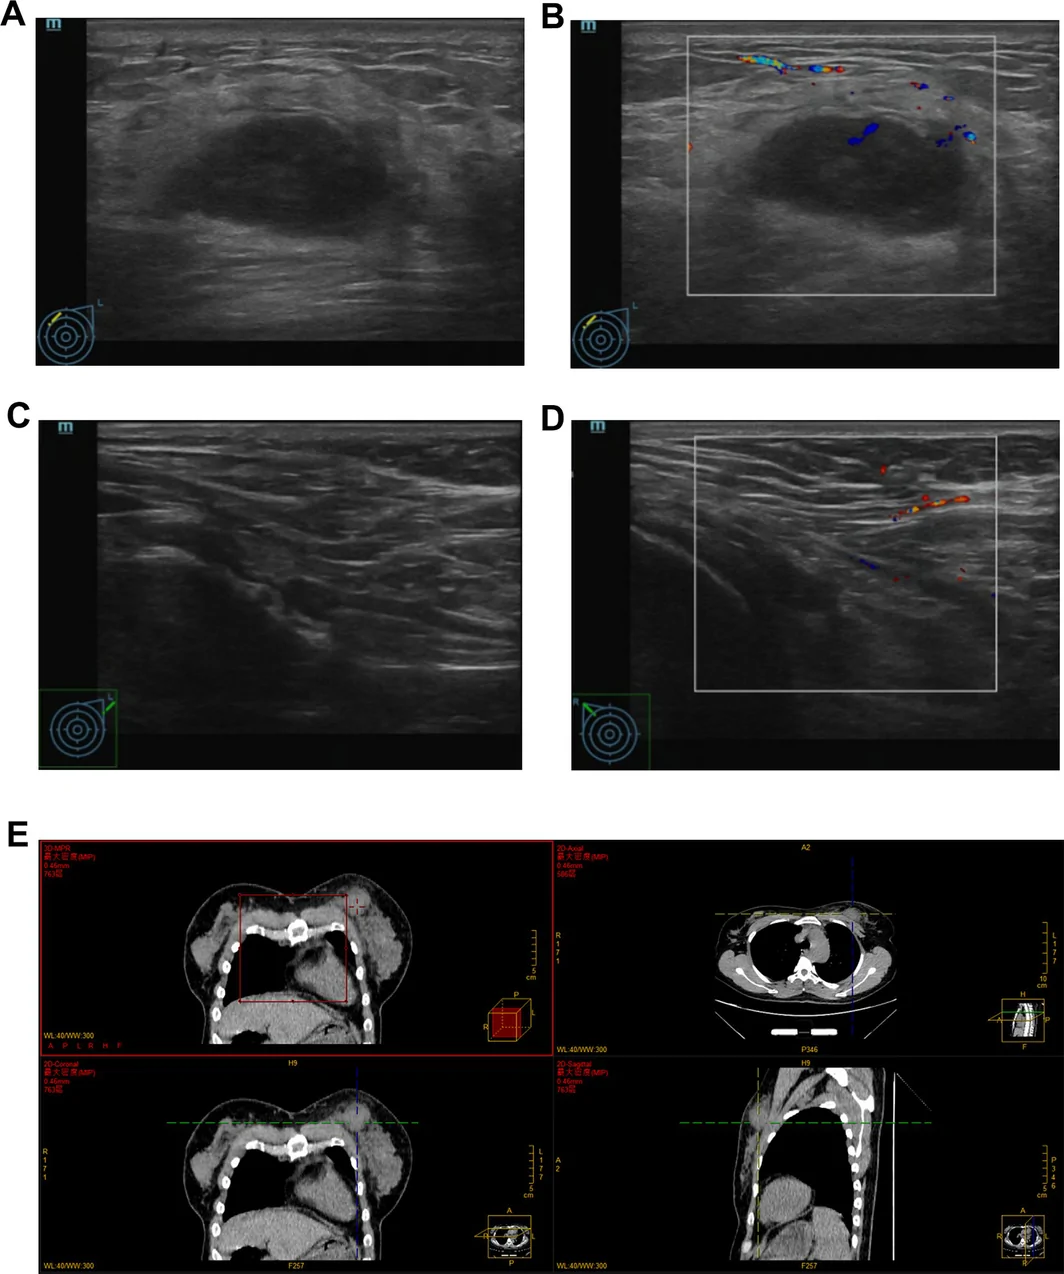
Initial breast color Doppler ultrasound (CDUS) and chest CT images obtained on March 6, 2020. (A–D) Breast CDUS images: Left breast mass (A), vascular blood flow signal of the mass (B), and bilateral axillary CDUS with no enlarged lymph nodes (C–D). (E) Multiplanar localized chest CT image of the left breast mass.
